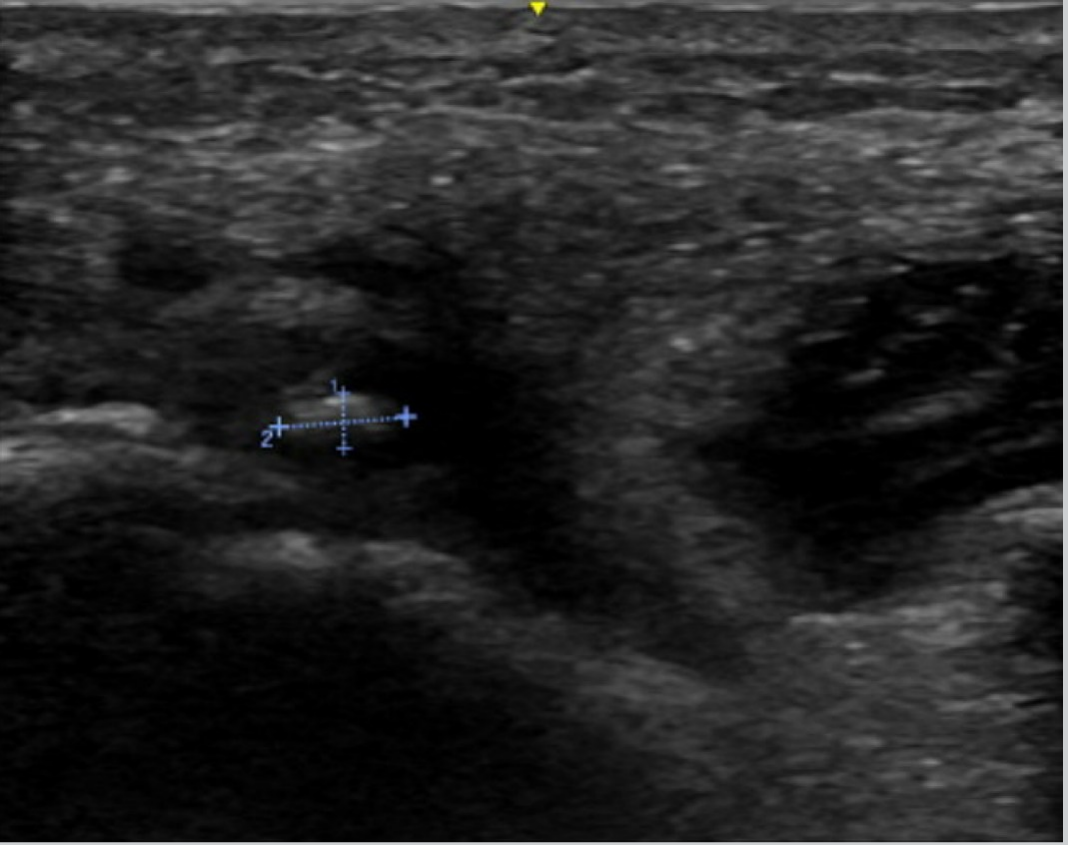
Left parotid duct sialolith (2 mm x 4.5 mm), transverse view on point of care ultrasound (POCUS) examination.
